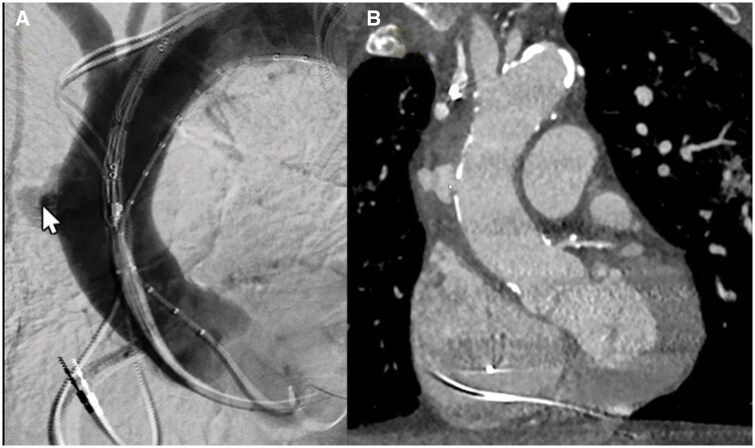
Imaging of Patient no. 6 with a Mycotic Pseudoaneurysm. (A) Intraoperative Angiography Showing the Lesion (Arrow). (B) Postoperative CTA Confirming Accurate Device Deployment and Exclusion. The Patient Died Four Months Later Due to Persistent Infection.
